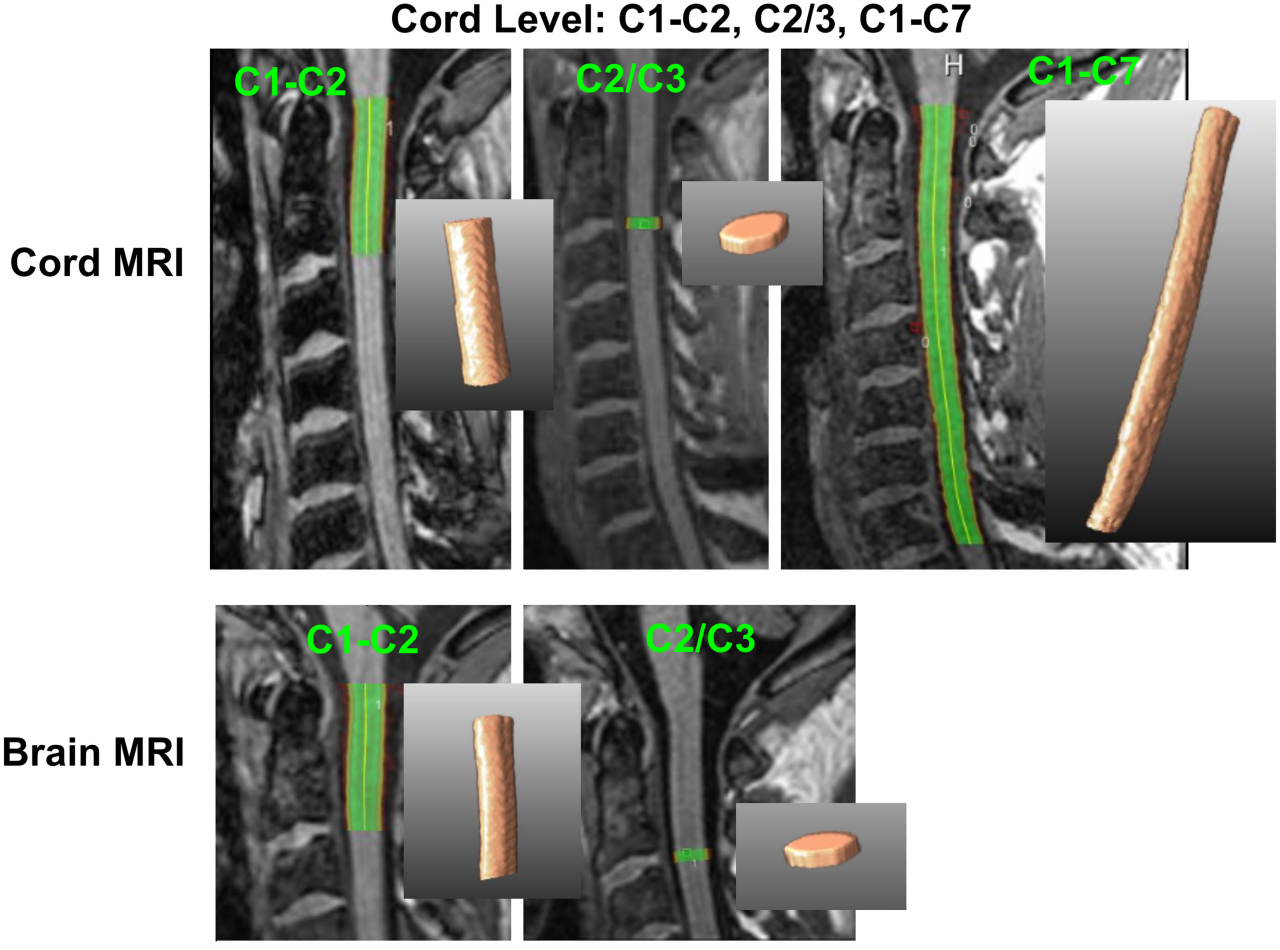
Definition of cervical cord level measurements on brain and cord MRI. C1–C2, upper cervical cord between C1 and C2 vertebra; C2/3, 3-mm slab at the C2/C3 disc level; C1–C7, entire cervical cord between the C1 and C7 vertebral level. 2D and 3D segmentations based on NQL are shown as examples.
